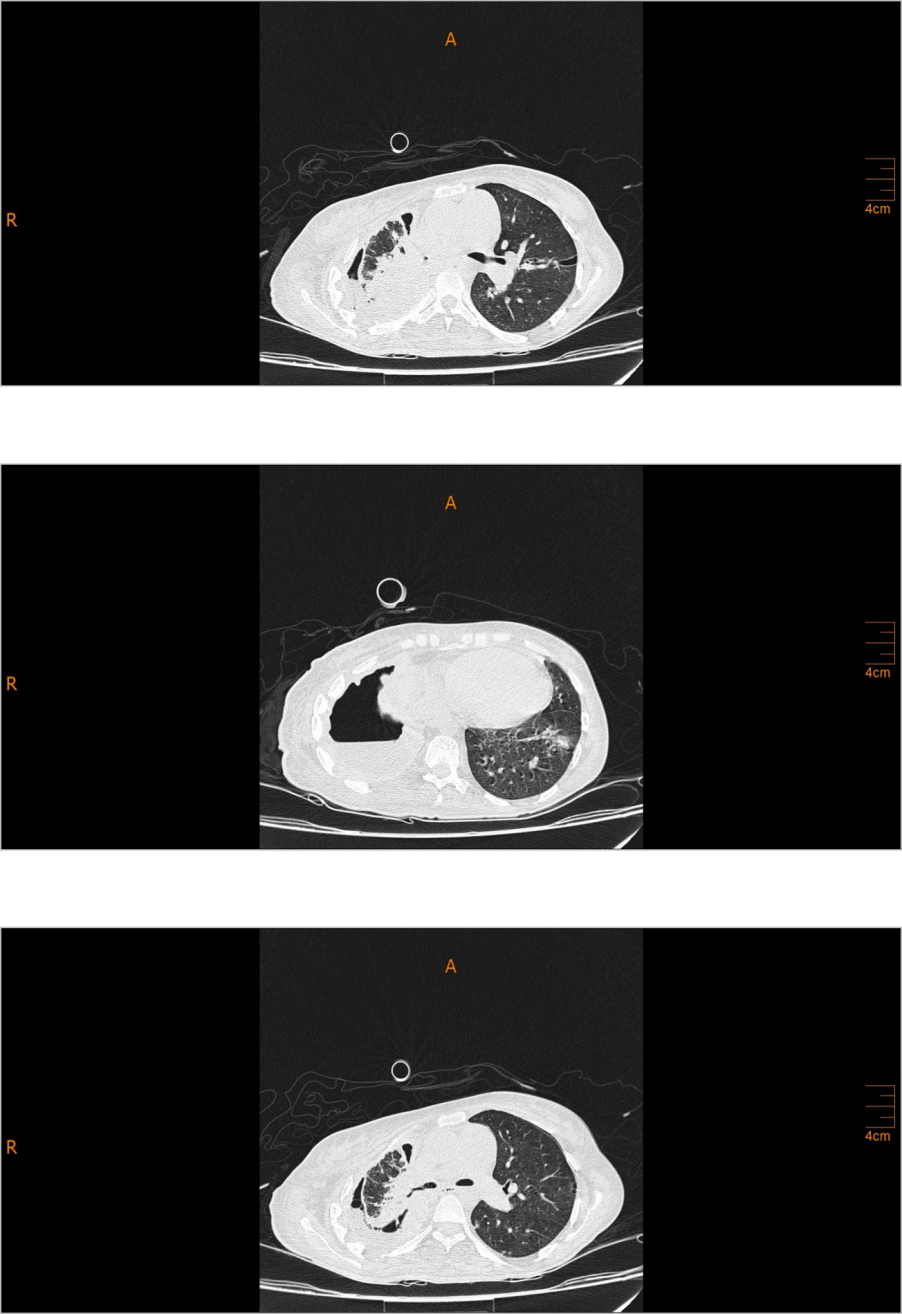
2022/08/10—spiral lung CT after surgery showing pleural effusion plus pyopneumothorax and consolidation causing the collapse of the RLL in the right hemithorax.
